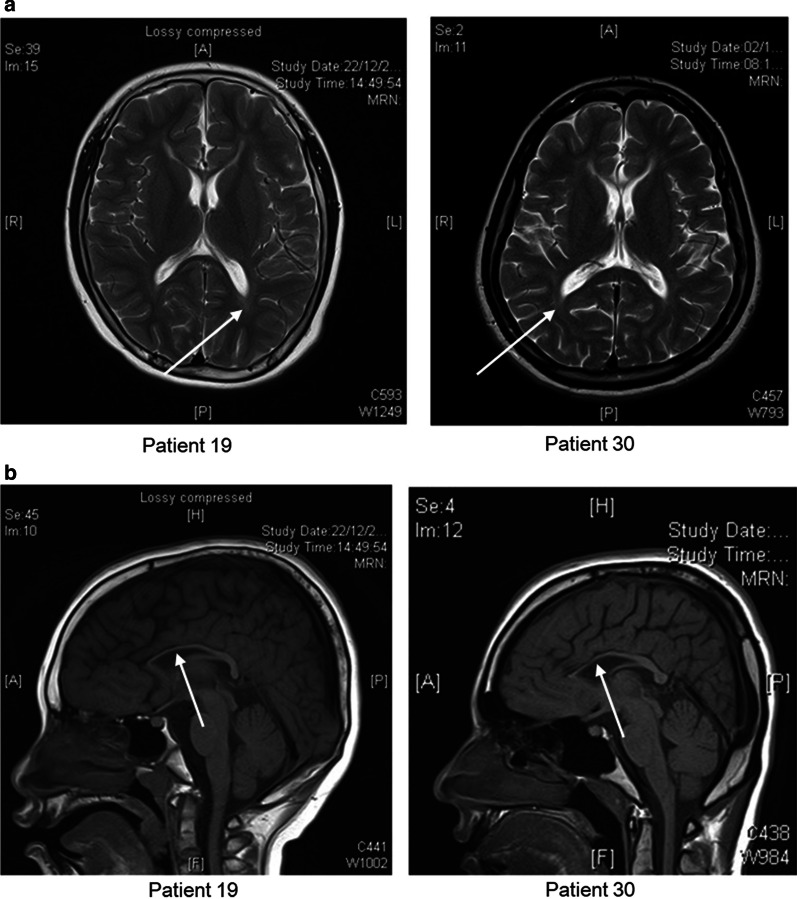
Brain Magnetic Resonance Imaging (MRI) of 2 patients with variants identified in *SPG11*. a Brain MRI of Patient 19 and 30 with periventricular white matter changes; b brain MRI of Patient 19 and 30 with thinning of corpus callosum. Arrows indicated the area with periventricular white matter changes and thinning of corpus callosum
